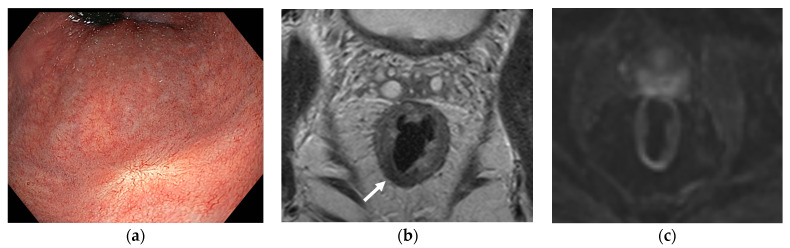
Clinical complete response (cCR) seen via (a) endoscopy with a typical white scar, (b) magnetic resonance imaging (MRI) with axial T2-weighted imaging with residual fibrosis (white arrow), and (c) diffusion-weighted MRI without high signal.
